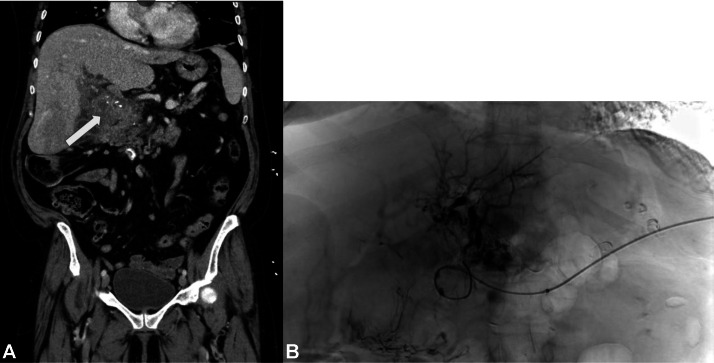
Portal cavernoma with collateralization. (A) Coronal CT portal venous phase image demonstrating PV thrombosis (white arrow) with numerous vascular collaterals, consistent with a portal cavernoma. A thin rim of contrast persists around the central filling defect, known as the polo mint sign. Medial to the arrow, calcifications of the porta hepatis can be visualized and (B) Splenic venogram showing extensive cavernous transformation of the PV with collateralization. Vertebral curve catheter and wires used could not successfully traverse the chronic PV thrombosis despite multiple attempts.
